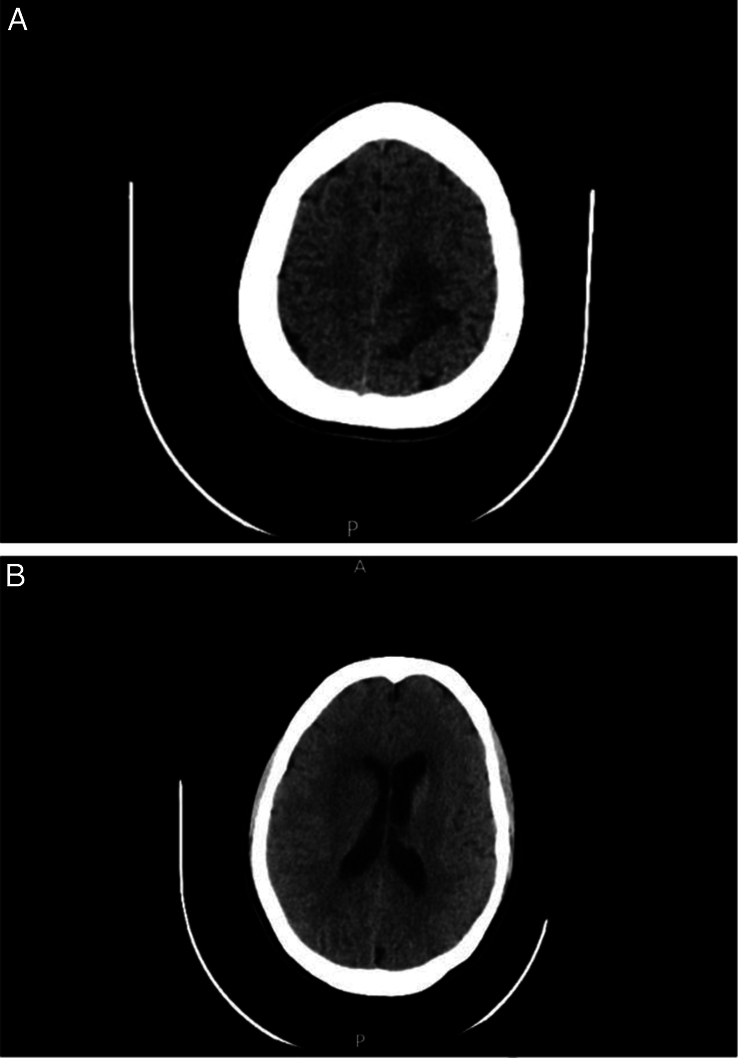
Cranial computed tomography shows few areas of brain parenchymal oedema in the left para-falci area (A) and the right periventricular area (B) with no intracranial haemorrhage, midline shift or bone lesions.
